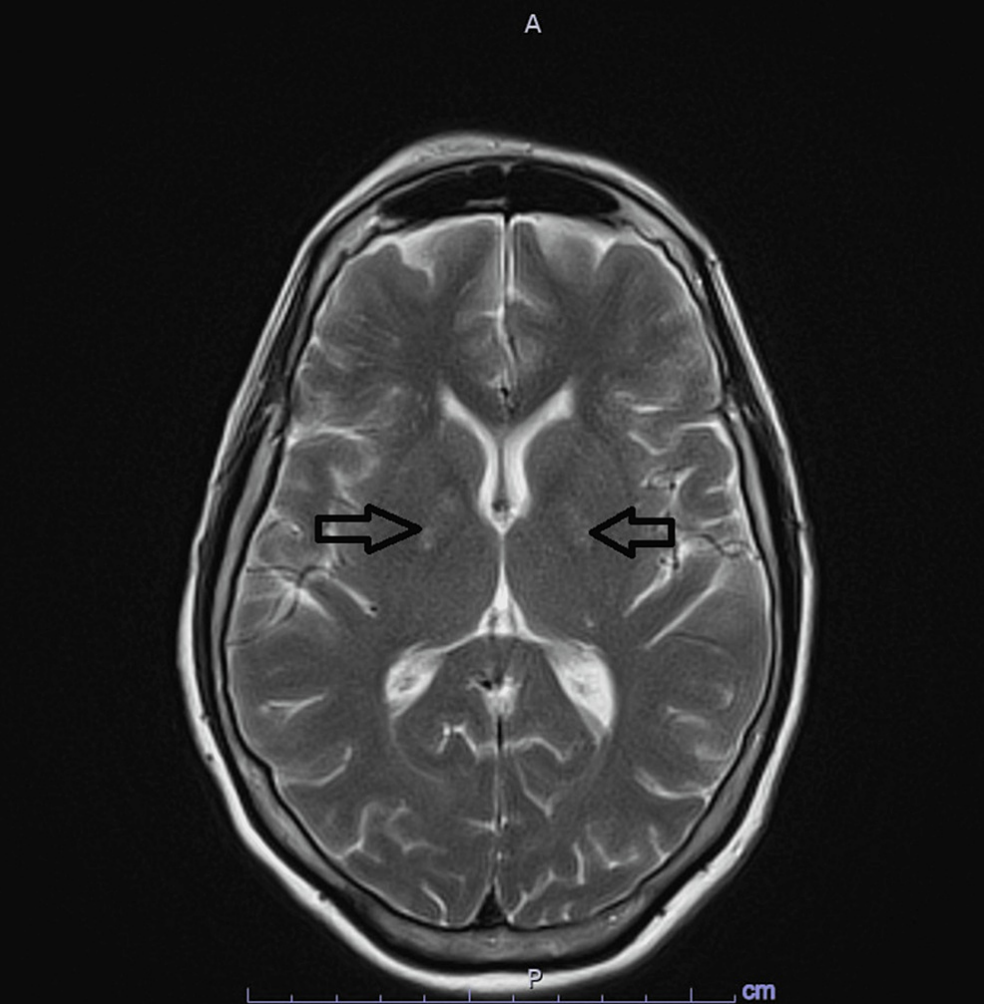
MRI Brain T2 MRI brain showing bilateral hyperintensity seen in globus pallidus (arrows).
